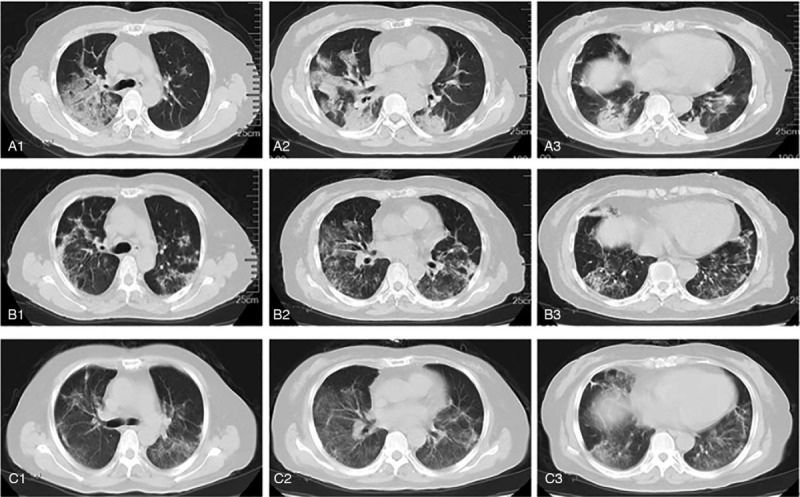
The typical CT images of the lung. A1–A3, CT images on day 2 indicate that there are lesions and mass density increasing shadow in both left and right lung. The ground-glass opacity, nonhomogeneous density, and air bronchus can be seen in the right lung. B1–B3, CT images on day 20 indicate the relief in both left and right lung. Only some Stripe shadow and small pieces of ground-glass opacity can be seen. C1–C3, CT images on day 25 indicate the further relief in both left and right lung. Most of the ground-glass opacity lightened, or even disappeared.
